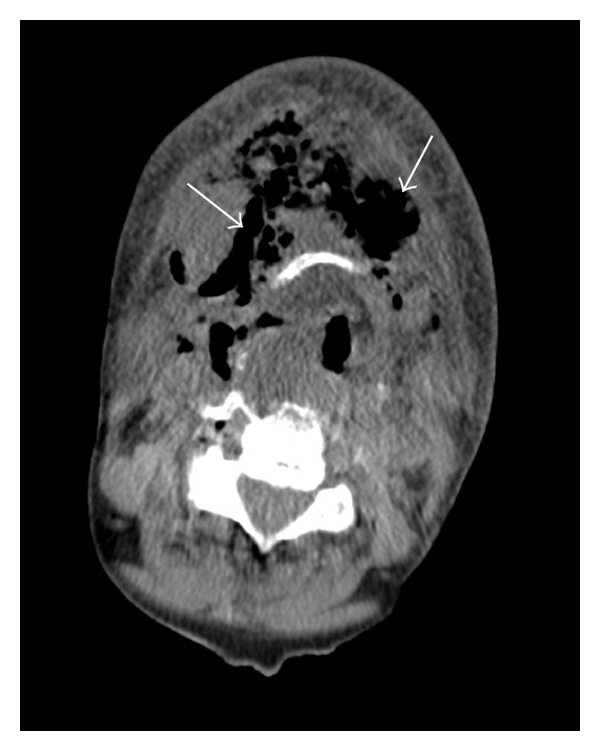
Axial CT image of patient preoperatively at level of hyoid. Note again presence of gas in anterior cervical space and stranding of fat suggestive of acute infection.
